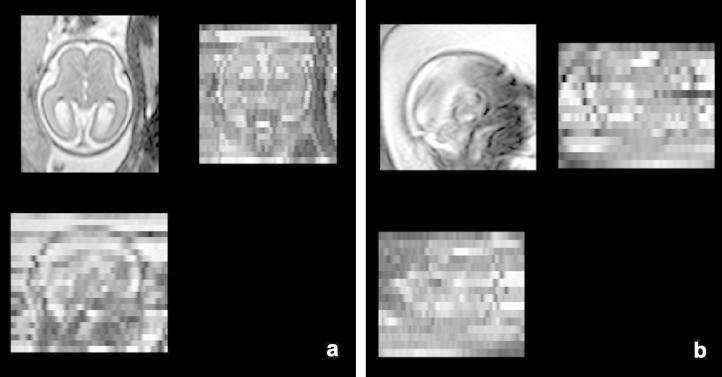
Motion artifacts in fetal MRI shown in three orthogonal plane views. (a) A stack acquired in axial direction exhibiting a smaller amount of motion. The individual images are of high quality but do not form a consistent representation. Structures can still be recognized in the through-plane direction. (b) A stack acquired in sagittal direction with heavy motion. Some slices are corrupted by motion artifacts. Structures cannot be recognized in the through-plane direction.
